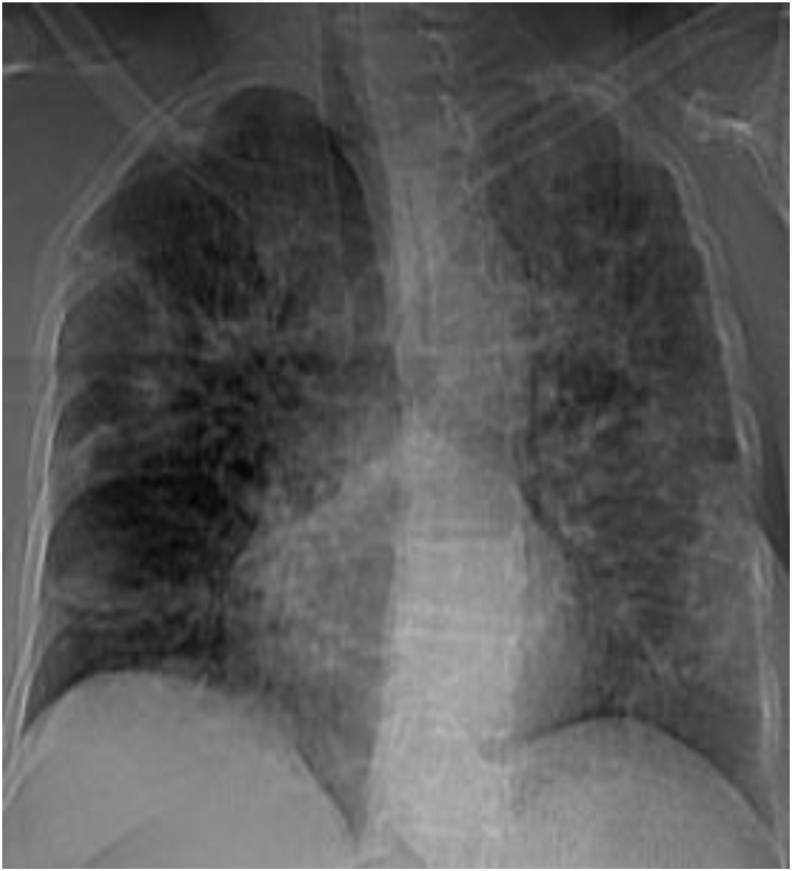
Frontal chest radiography showing bilateral air space consolidations in a Covid-19 patient.
